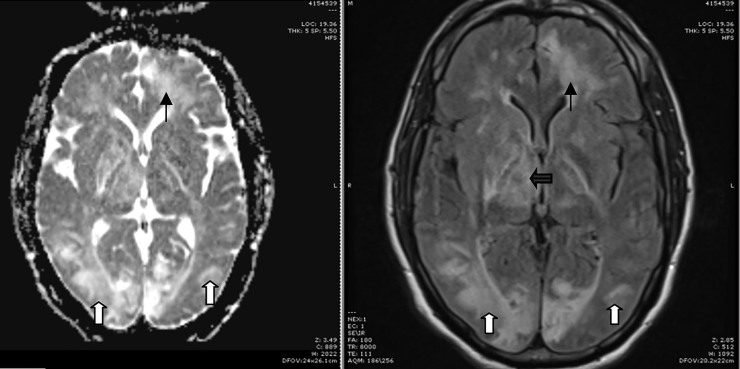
MRI Left: Diffusion imaging showing increased signal in the bilateral occipital lobes (white arrows) and left frontal lobe (black arrow). MRI Right: T2 FLAIR imaging showing correlating hyperintensities in bilateral occipital lobes (white arrows), left frontal lobe (black arrow) and right periventricular area (black bold arrow). *MRI*, magnetic resonance imaging; *FLAIR*, fluid attenuation inversion recovery
